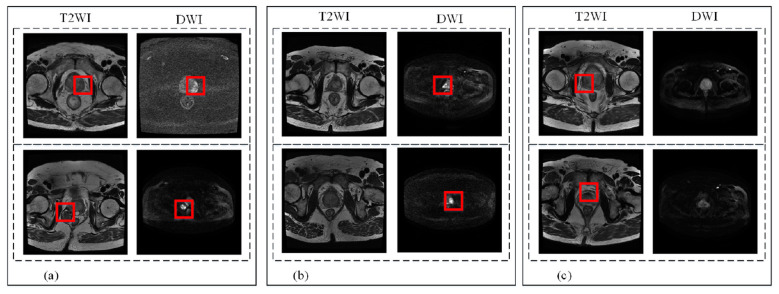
Imaging manifestations of prostate cancer (PCa) in dual-modal bpMRI. (a) Cancer appears in both modes. (b) Manifestation in cancer in DWI. (c) Manifestation in cancer T2WI.
